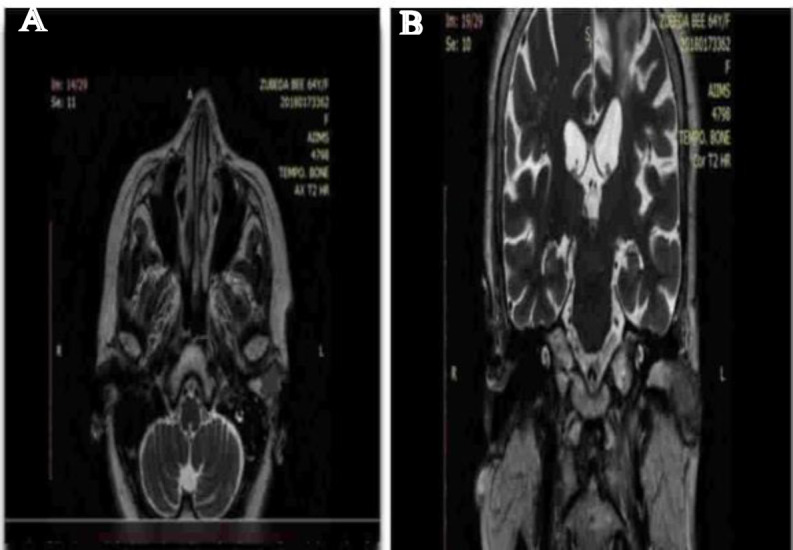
Magnetic Resonance Imaging of Temporal bone revealed a 1.5 x 2.9 x 1.2 cm sized lesion in the left EAC involving both the cartilaginous and bony part
